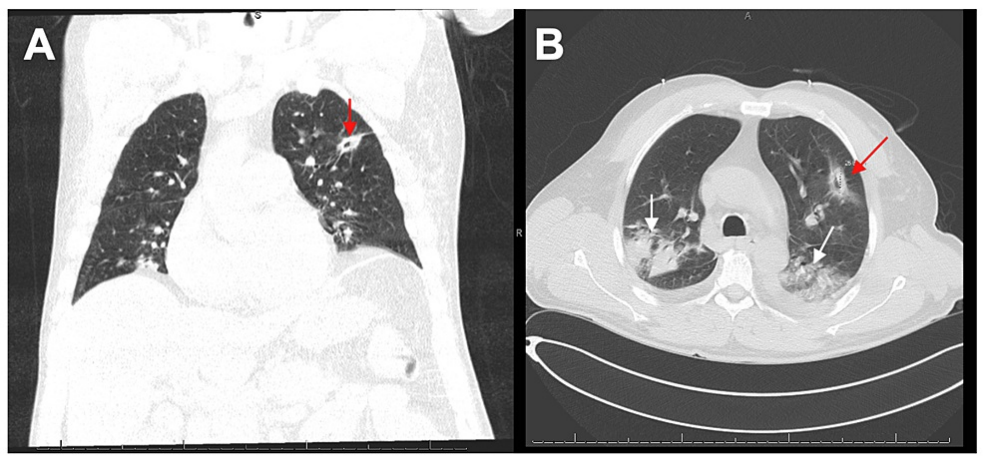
CT Chest without Contrast (A, B) Large multifocal airspace consolidations in the bilateral upper and lower lobes (white arrows). Pulmonary nodule with a central cavitary lesion seen on the left upper lobe (red arrows). Development of right upper lobe and bilateral lower lobe pneumonia.
